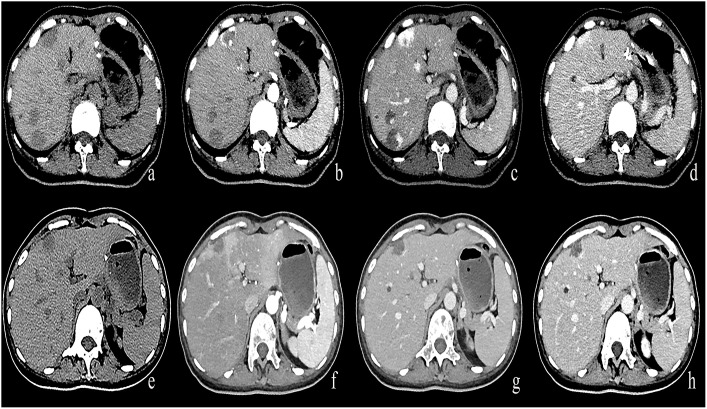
(A–D) A round low-density mass in the left inner lobe of the liver was shown in the plain CT. The lesions demonstrate peripheral nodular discontinuous enhancement in the arterial phase and centripetal filling in the portal and delayed phases. (E–H) After treatment with Bevacizumab, the lesions showed insignificant enhancement.
